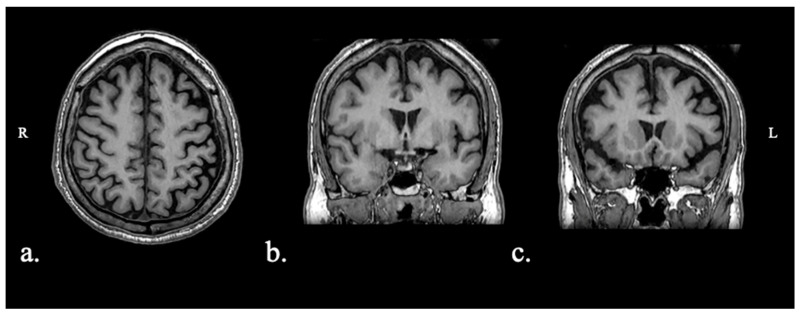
Patient IZS MRI scan: (a) axial view with slightly asymmetrical (L > R) parietal atrophy; (b) coronal view with bilateral atrophy of amygdala; (c) coronal view with asymmetrical (L > R) frontal atrophy.
